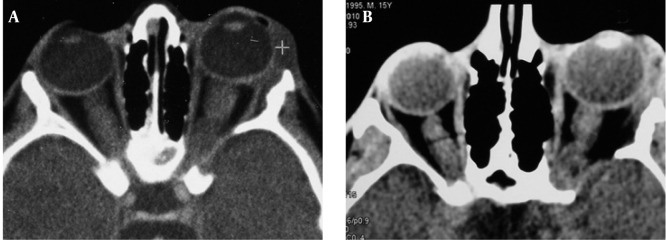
A, CT scans of the orbit at the age of 3 years and; B, The age of 15 years show soft tissue density in supralateral portion of left orbit adjacent to the globe. Thick optic nerve and bony defect in the left posterior and lateral wall of the orbit are observed.
